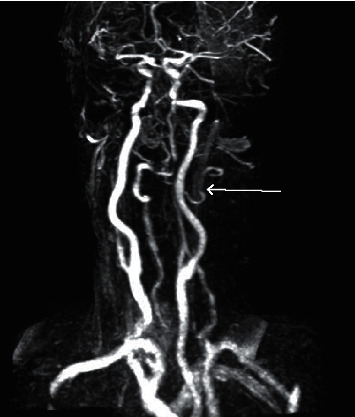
CTA showing left vertebral artery dissection.
